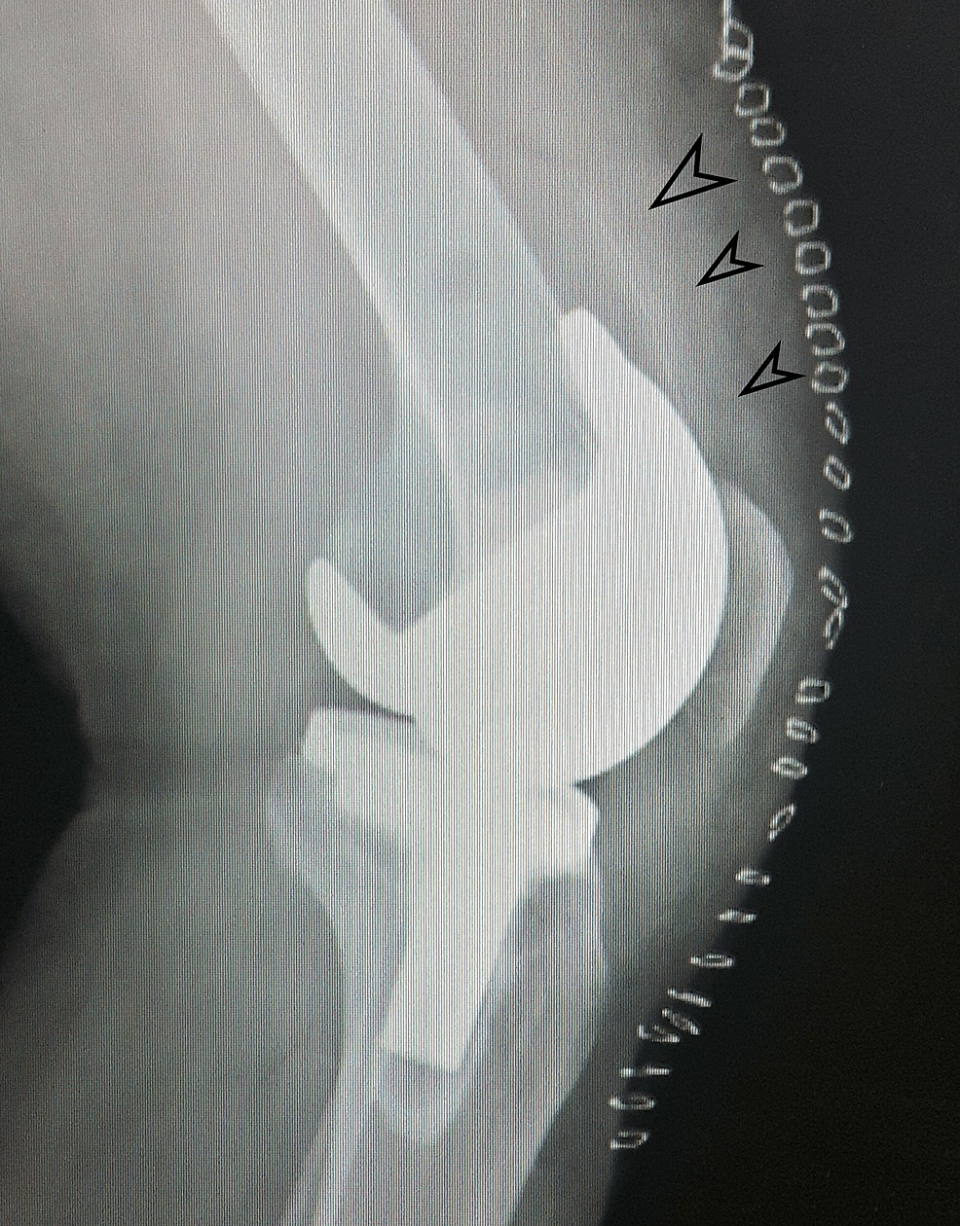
Post-operative radiograph of the left knee (lateral view) Post-operative radiograph of the left knee in lateral view delineating the broken drain piece with multiple black arrows.
